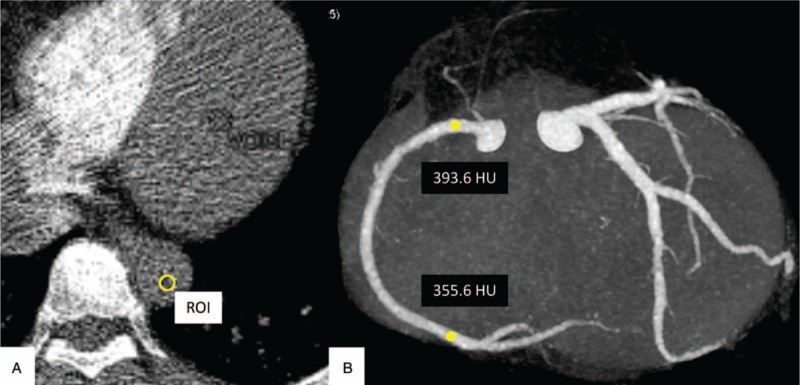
Example case of a 46-year-old woman with a dorsally placed ROI. CT values of the proximal and the distal RCA were 393.6 HU and 355.6 HU, respectively. Contrast arrival time was 18.65 seconds. CT = computed tomography, HU = Hounsfield units, RCA = right coronary arteries, ROI = region of interest.
